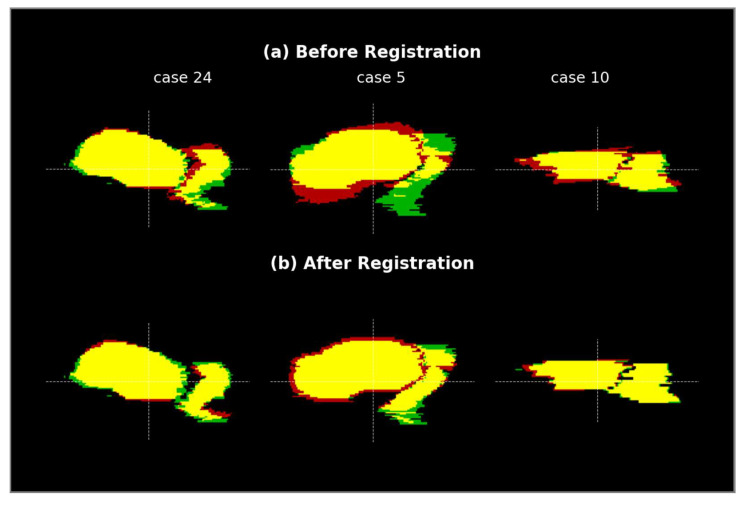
Organ alignment before and after registration in sagittal view. Red denotes the moving CT region, green indicates the fixed CBCT, and yellow highlights their overlapping area.
